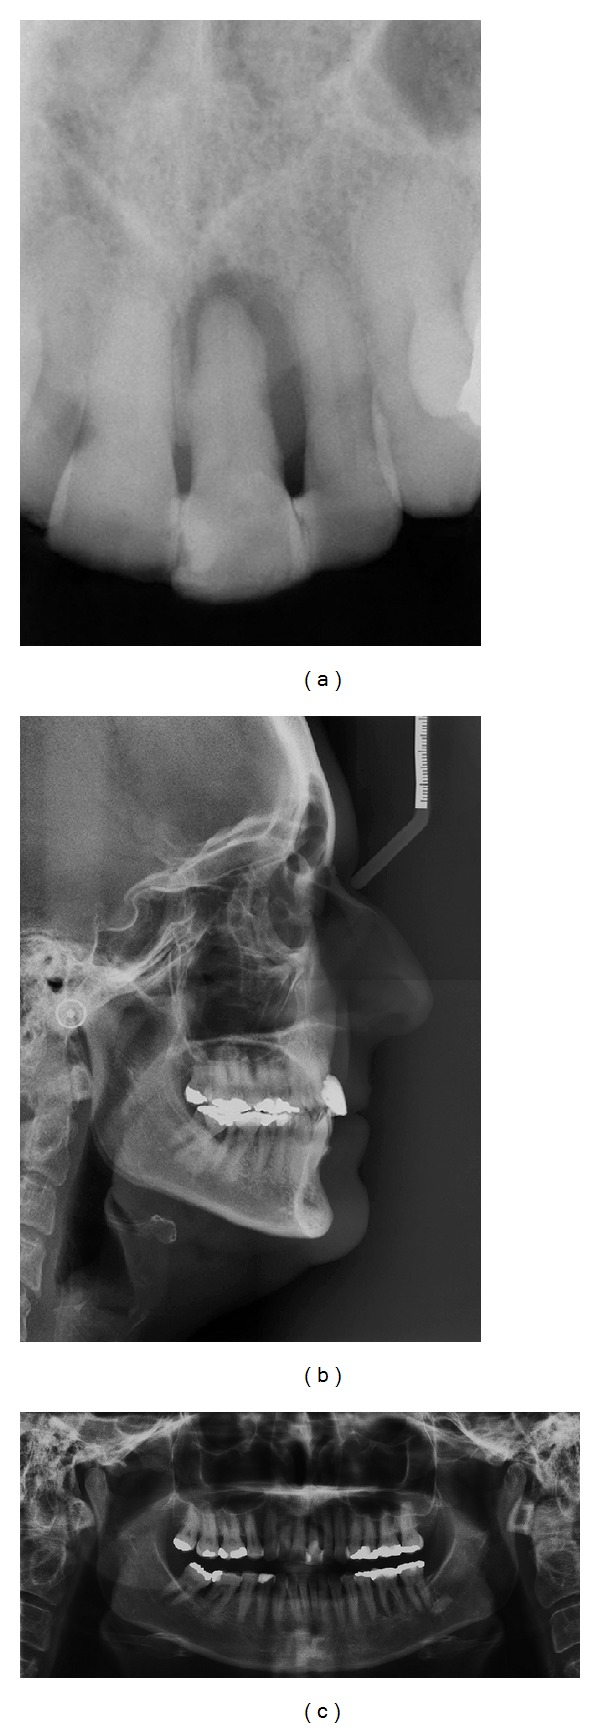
Pretreatment periapical, cephalometric, and panoramic radiographs.
